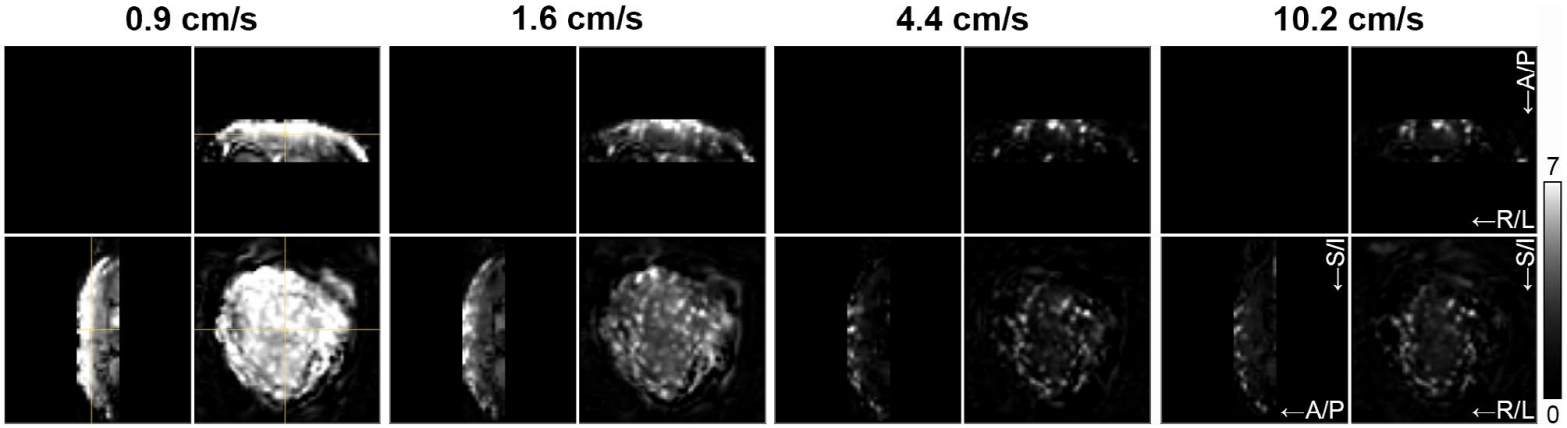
Variation of cutoff velocity. Placental perfusion images obtained with VS‐ASL MRI using four different cutoff velocities (0.9, 1.6, 4.4, and 10.2 cm/s) in a pregnant volunteer (Subject 8 in Table 1). Orthogonal slices (spatial resolution: 4 × 4 × 4 mm^3^) are shown. The cross‐hair in the left images indicates the intersection of the slices. Images have equal LUT scaling. The grayscale bar indicates PWS [%]. A/P, anterior‐posterior; R/L, right‐left; S/I: superior‐inferior
